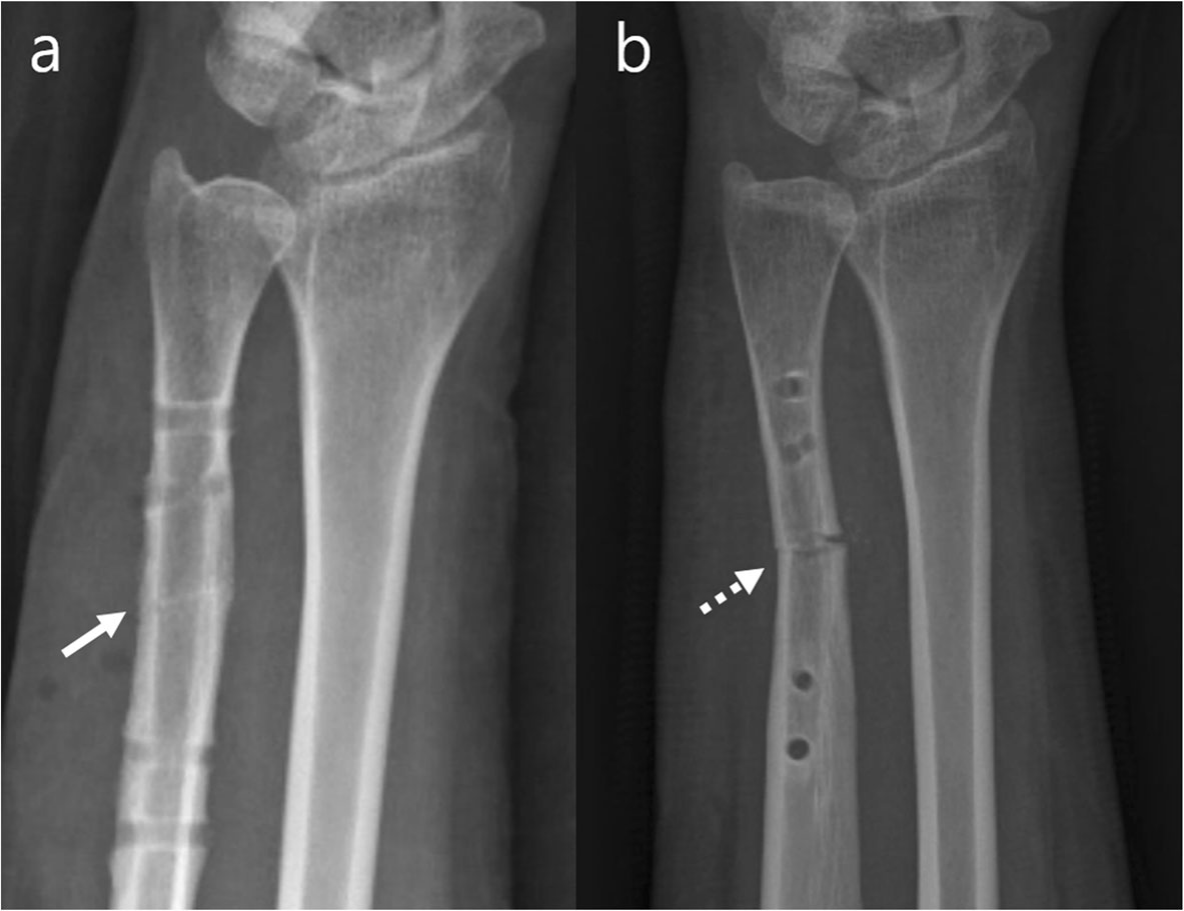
Radiograph of the case with a osteotomy trace (solid arrow) and b refracture after plate removal (dot arrow)
